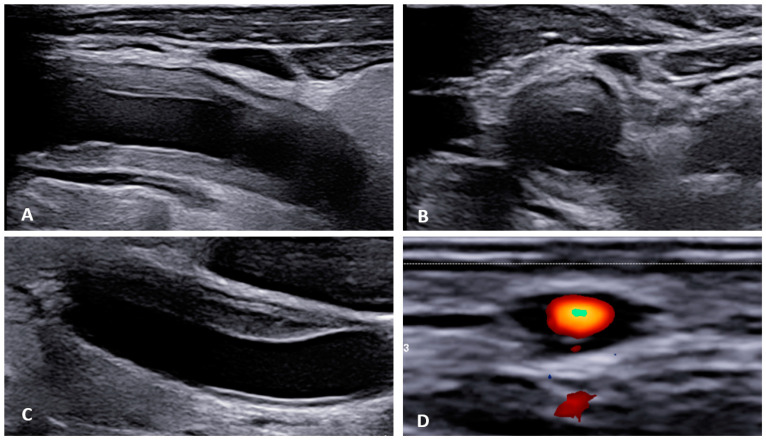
Ultrasonographic findings in patients with large vessel vasculitis: (A,B): B-mode US with the finding of concentric carotid intima-media thickening in a patient with Takayasu’s arteritis ((A) longitudinal plane, (B) transversal plane). (C). B-mode US: carotid intima-media thickening in a patient with giant cell arteritis. (D): Power Doppler US in a patient with temporal arteritis (typical “HALO” sign). Courtesy of Dr. Konstantinos Triantafyllias, Rheumatology Center, Rhineland-Palatinate.
